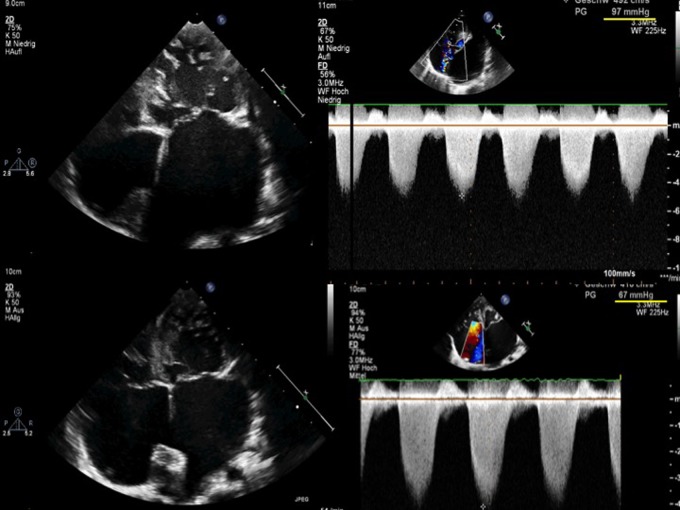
Restrictive cardiomyopathy (RCM), effects of a restrictive interatrial communication. Two-dimensional (2-D) echocardiography (apical four-chamber view) and continuous wave (CW)-Doppler measurements (tricuspid regurgitation, TR) of a toddler with RCM referred for heart transplantation (HTx); the 2-D echocardiography shows massive left atrial enlargement associated with a ‘out-of-proportional’ left atrial pressure related pulmonary hypertension at a suprasystemic level (systolic pressure gradient of 97 mm Hg, upper panel). The creation of a restrictive atrial septum defect, by placing a stent within the perforated atrial septum, led to a decreased left atrial dimension/pressure as well as reduced pulmonary artery pressures (lower panel). Placement of an assist device could be avoided, a successful HTx performed.
